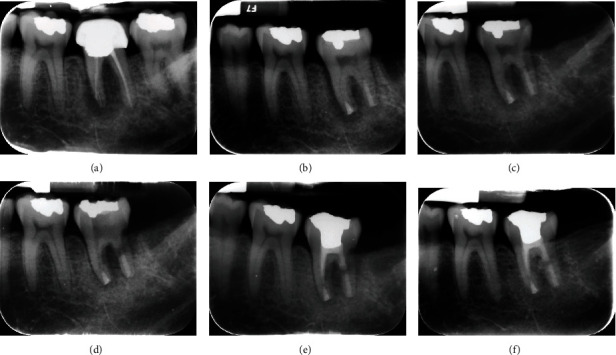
Mandibular left second molar with large periapical radiolucency: (a) mandibular left second molar with inappropriate previous root canal therapy, intracanal broken instruments, large periapical radiolucency, and furcation involvement; (b) former mandibular second molar was diagnosed hopeless and thus was removed whereas the mandibular left third molar was soundly extracted, and root-end cavities were cautiously prepared, carefully sealed using calcium enriched mixture (CEM) cement, and transplanted as a replacement for the mandibular left second molar; (c) in the 3-month follow-up, relative healing of periradicular bone was evident; however, radiolucencies in the mesial periapical region and furcation persisted, and signs of external inflammatory root resorption (EIRR) appeared on the distal root; (d) after the 6-month follow-up from the first treatment session and persistence of EIRR signs, the coronal restoration was removed, root canals were prepared, canals were medicated with a new combination of triple antibiotics (PMC), and the coronal cavity was sealed with Zonalin™; (e) after 3 weeks from the previous appointment, relative bone healing and complete arrest in EIRR progression were seen. Root canals were then filled with CEM cement, and the coronal cavity was restored using amalgam; (f) in the 2-year follow-up, thorough bone healing, disappearance of periapical and furcation radiolucency, and arrest/treatment of IERR were observed.
